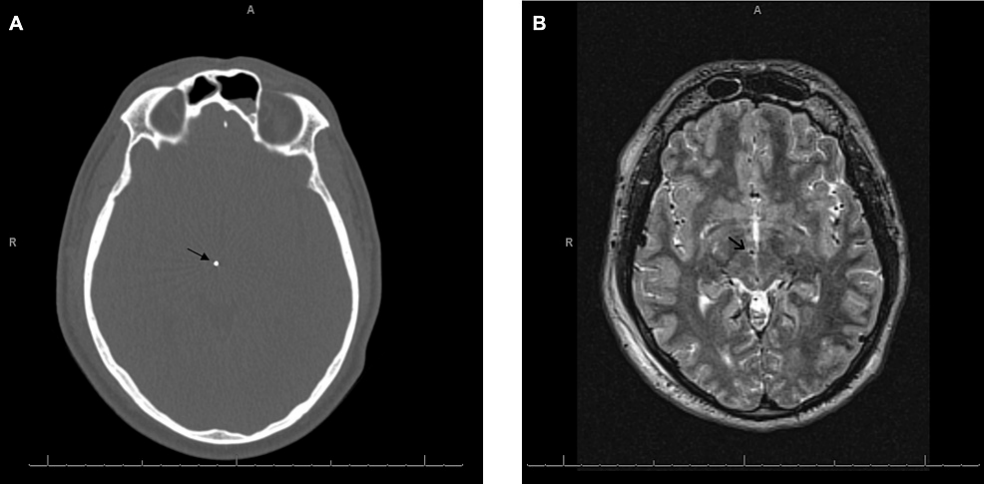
(A) CT and (B) MRI scans post-DBS surgery. The location of electrodes in the posterior inferior hypothalamus is shown with a black arrow. DBS: Deep brain stimulation
